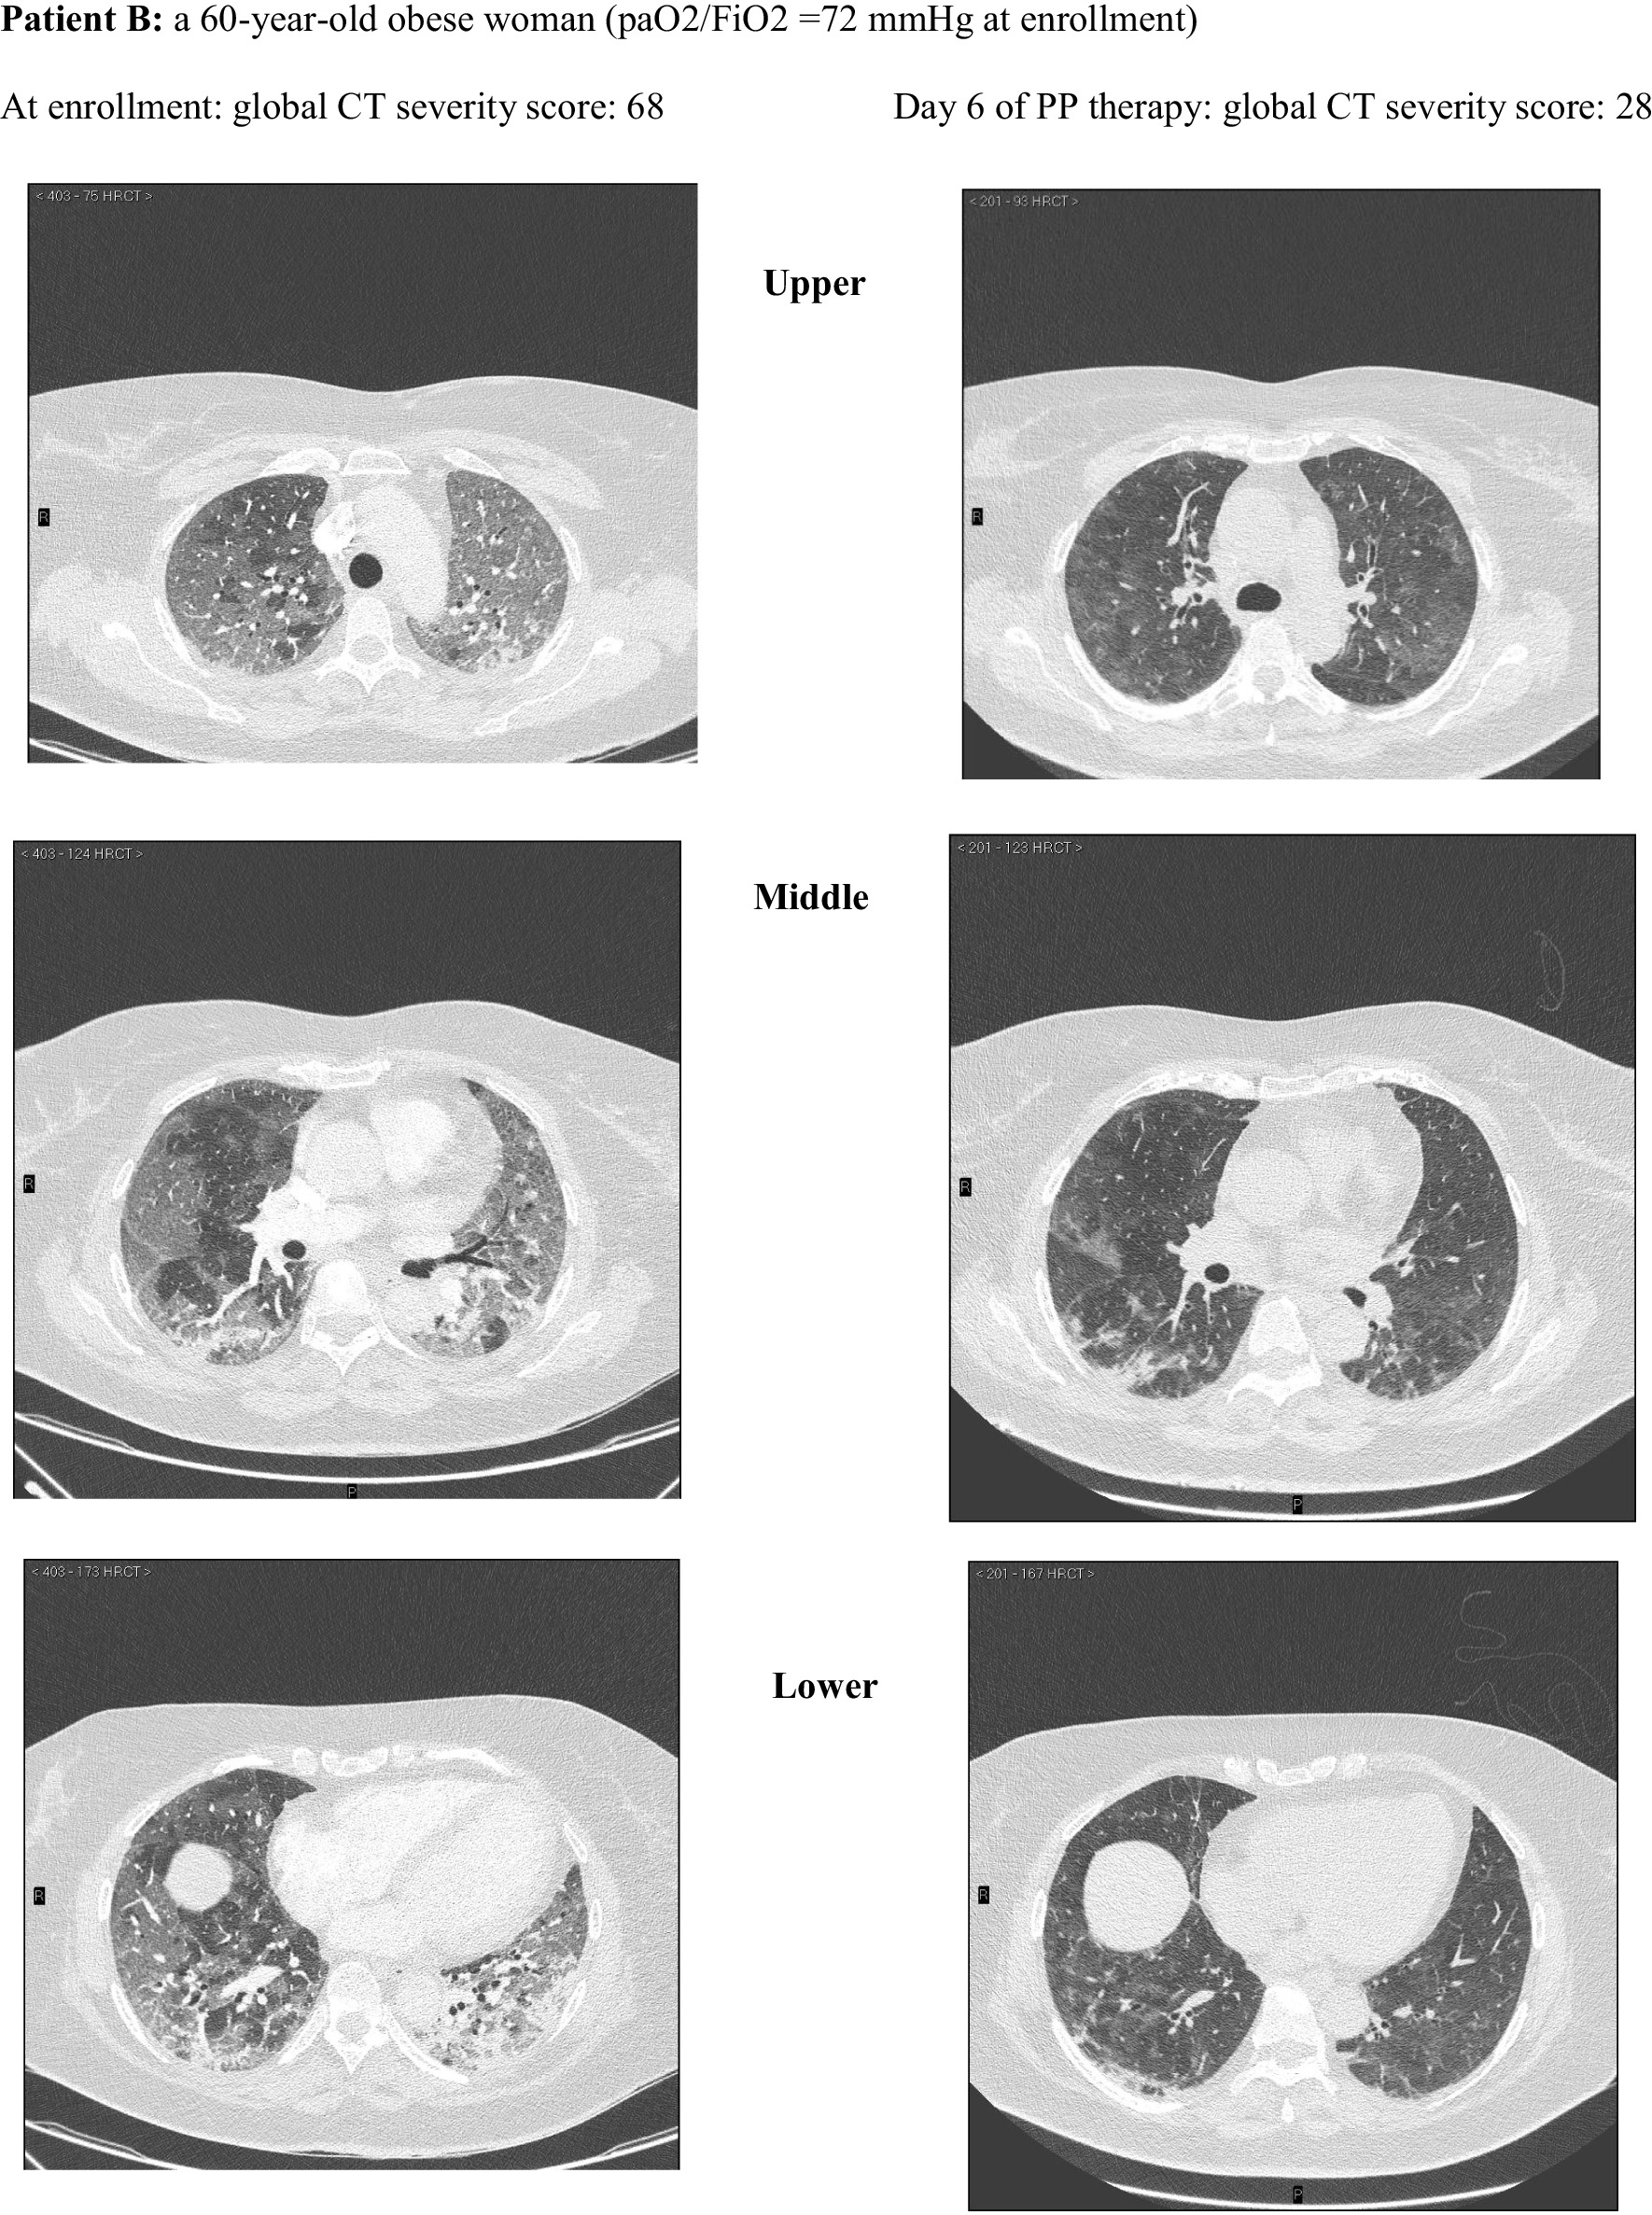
Extent and nature of lung parenchymal involvement at three levels (upper, middle, lower) were quantified according to the score validated by Salaffi et al. (score range 0–96). Two cases with pre/post-treatment CT scans are provided below
